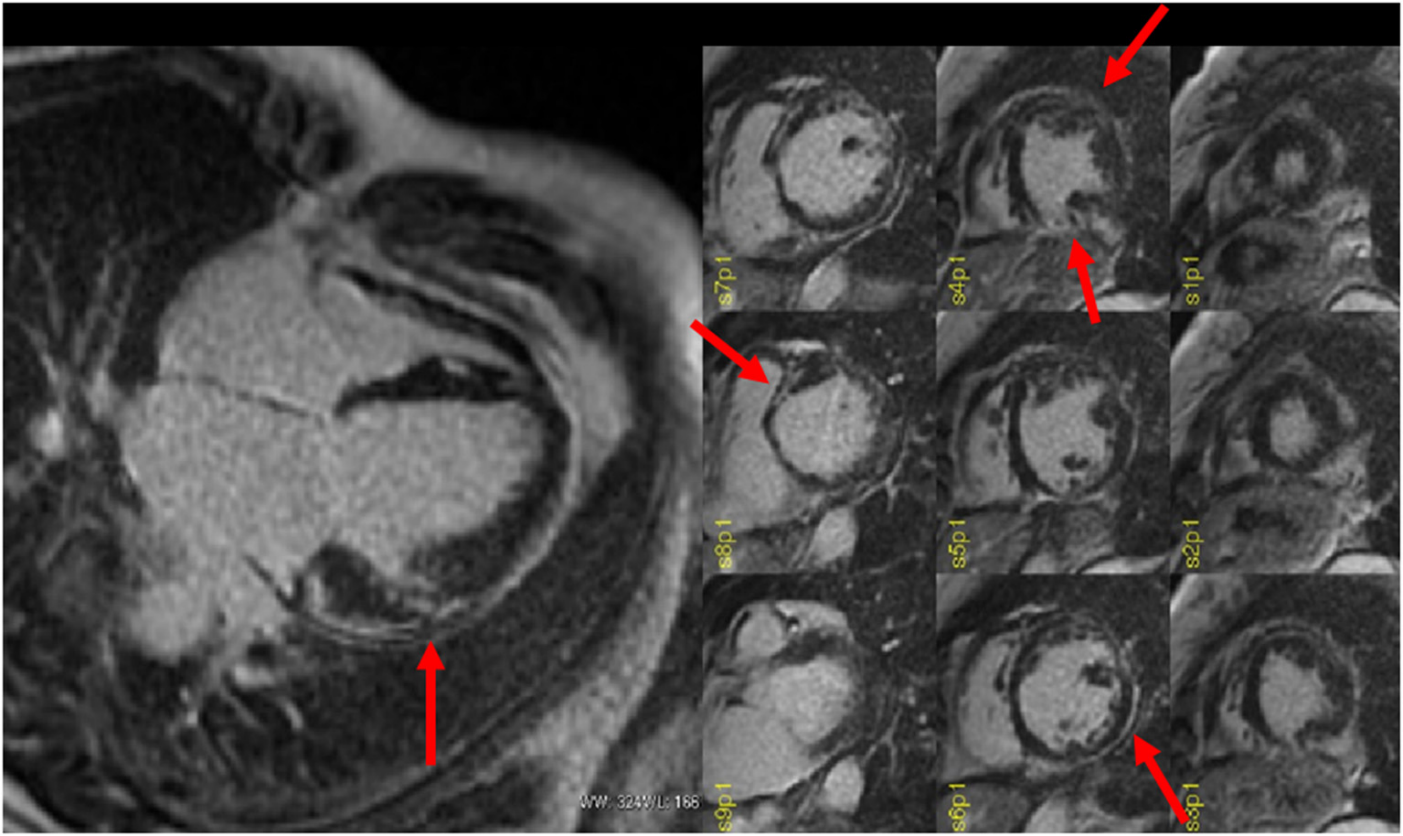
Late gadolinium enhancement (LGE) MRI images obtained in a patient with a previous history of myocarditis and with a recent history of hemodynamically stable sustained ventricular tachycardia (VT) scheduled for ablation procedure. The four-chamber (left) view shows the presence of a focal LGE area on the mid-ventricular segment of the antero-lateral wall (almost transmural). Short-axis images (right) show the real extent of LGE that also encompasses the inferior and lateral left ventricular (LV) walls (sub-epicardial), the antero-septal wall (midwall), and the distal anterior segment. Courtesy of Dr. Giovanni Donato Aquaro, Fondazione Toscana Gabriele Monasterio, Pisa, Italy.
